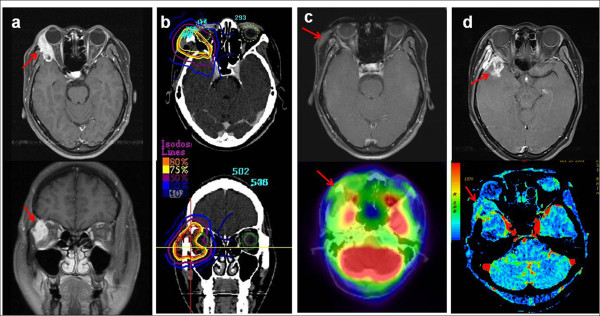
Radiation retinopathy, neovascular glaucoma and temporal lobe necrosis after SBRT. This patient was a 36-year-old female with adenoid cystic carcinoma (ACC) of the right lacrimal gland with intraorbital invasion (a). She underwent right orbitotomy and neck dissection followed by EBRT at 45 Gy and SBRT at 25 Gy in 5 fractions (b) and achieved CR in the response evaluation (c). Two years after SBRT, a cataract operation (Phaco + PCL) of the right eye was performed. She experienced progressive vision loss in the right eye and was diagnosed with radiation retinopathy and neovascular glaucoma in an ophthalmologic exam 30 months after SBRT. Follow-up serial perfusion CT and functional MRI of the brain indicated right temporal lobe necrosis of the brain (d).
